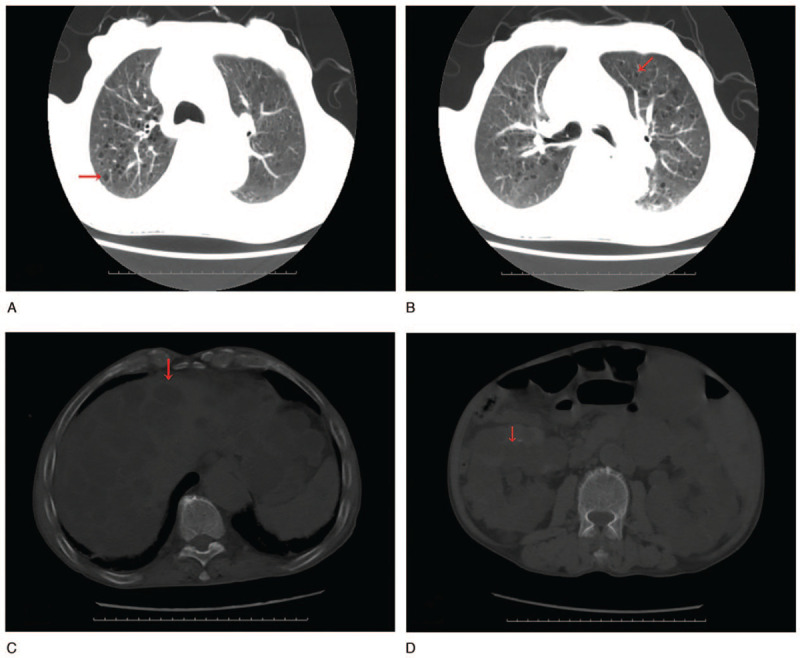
Computed tomography scans show multiple cysts (arrows) in the lungs, liver, and kidneys, with a very small proportion of normal residual parenchyma.
